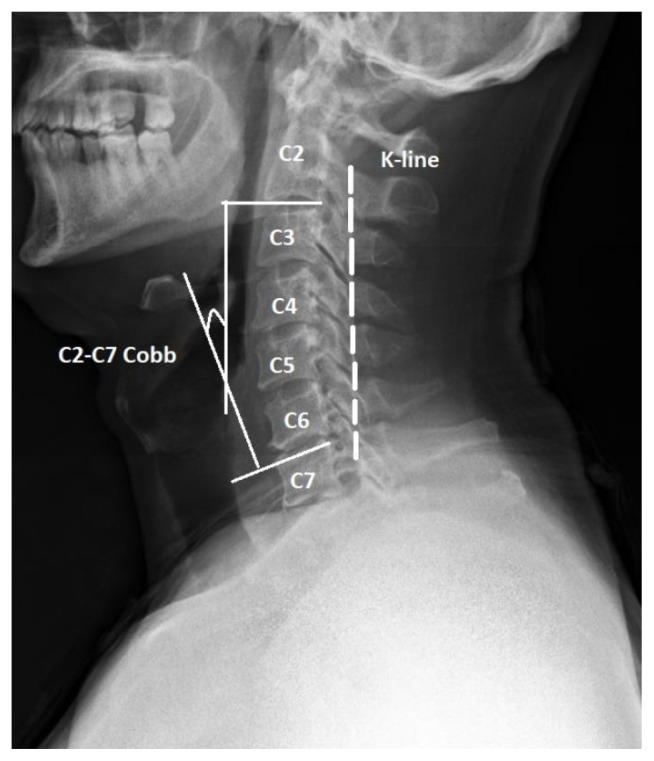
Lateralc-spine x-ray. We calculated both the Cobblor doticangle (LA) (angle between lower C2 end plate and superior C7 endplate) and the kyphosis line (K-line) (in the neutral position of the neck drawn between midpoints of the spinal canal C2 to C7).
